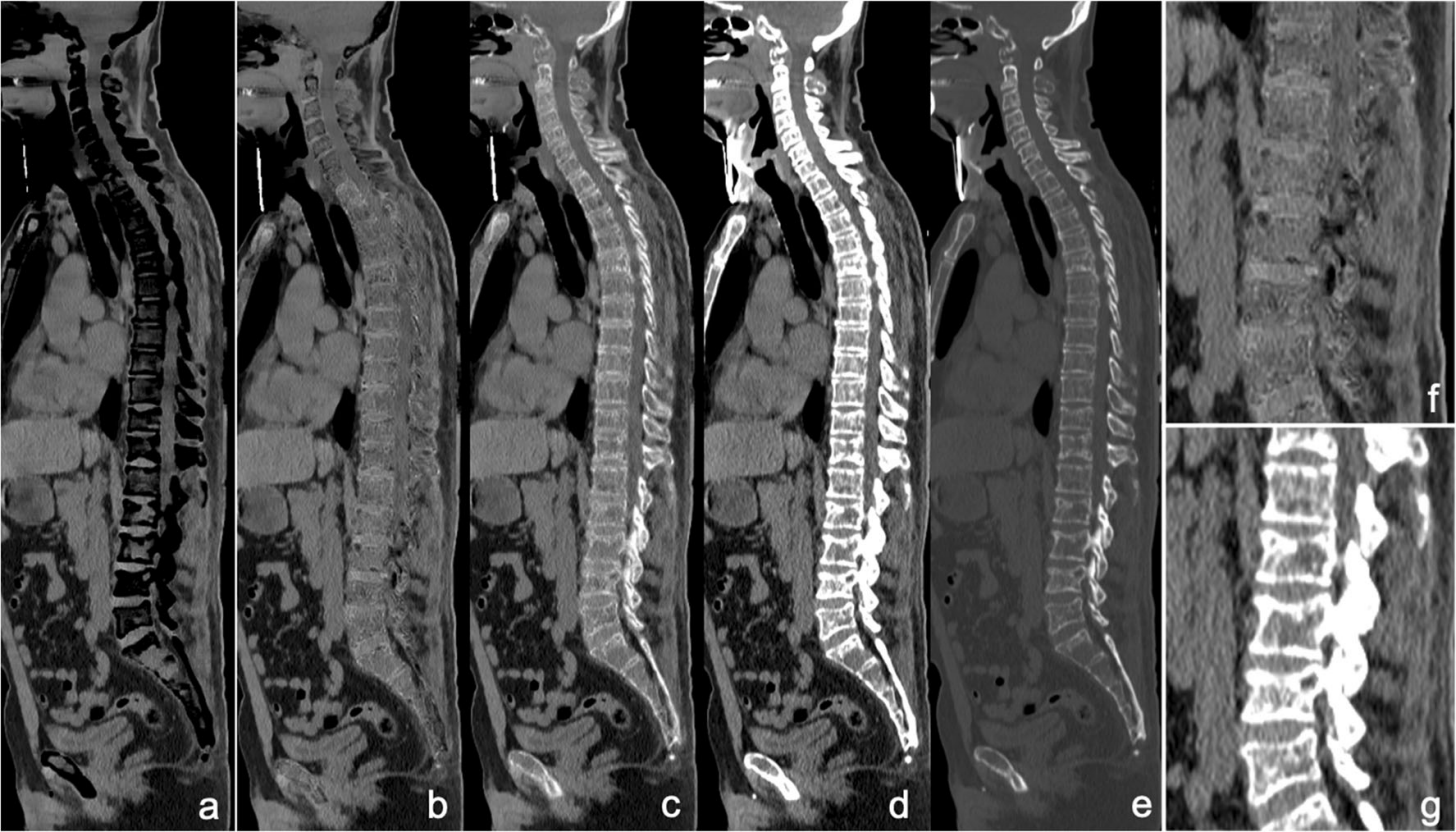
Patient with diffuse infiltration; a CaSupp index 25; b CaSupp index 65; c CaSupp index 95; d conventional CT soft tissue kernel; e conventional CT sharp kernel; f, g magnification of b and d for L1-5
